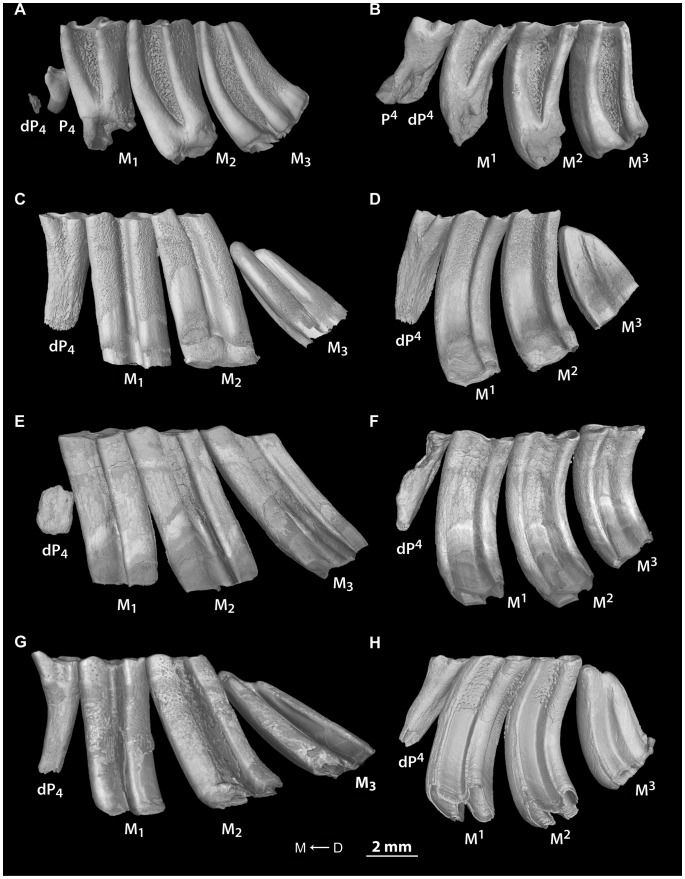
Lower and upper cheek tooth rows of each extant ctenodactylid in lateral view. X-ray synchrotron microtomographic 3D renderings of A–B, *Pectinator spekei* (MNHN-CG1893-226), C–D, *Ctenodactylus gundi* (MNHN-CG1986-255), E–F, *Felovia vae* (MNHN-CG1989-22) and G–H, *Massoutiera mzabi* (MNHN-CG1955-2). D → M stands for distal to mesial direction.
